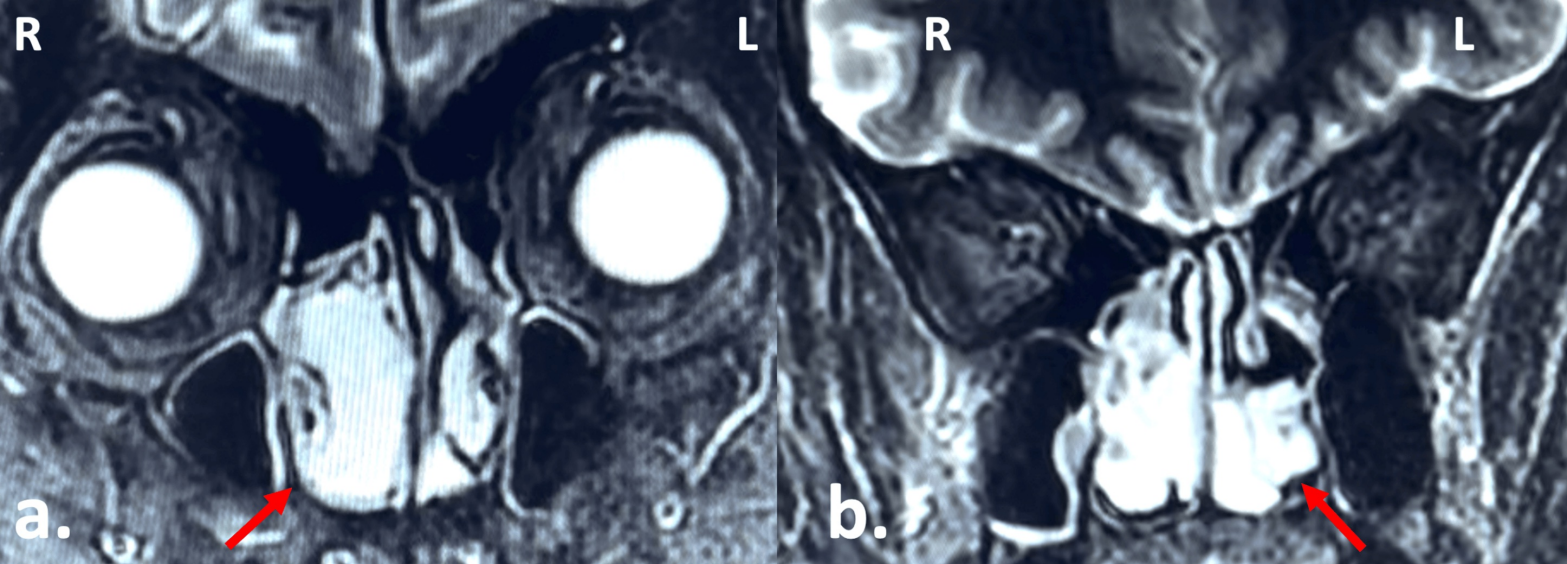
Magnetic resonance imaging of the head with gadolinium (T2-sequence; coronal view) revealed similar enhancement of the pathologic tissue (indicated by the red arrows) compared to that of the inferior turbinates. (a) Anteriorly; (b) posteriorly; R: right; L: left.
